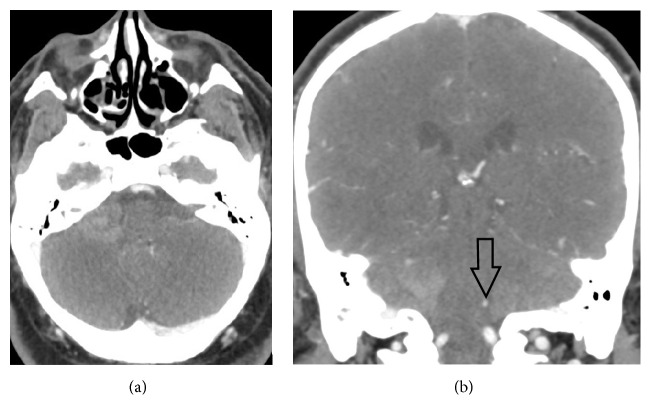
Axial (a) and coronal (b) CT angiography (CTA) of the head in the arterial phase demonstrates unchanged hemorrhage in the region inferior right cerebellar peduncle, likely intraparenchymal, with extension into the fourth ventricle without evidence of aneurysm or vascular malformation. The right posterior inferior cerebellar artery (PICA) is not well seen (arrow in (b) indicates the normal left PICA) and may be compressed by surrounding hemorrhage, involved with vasospasm or thrombosed.
